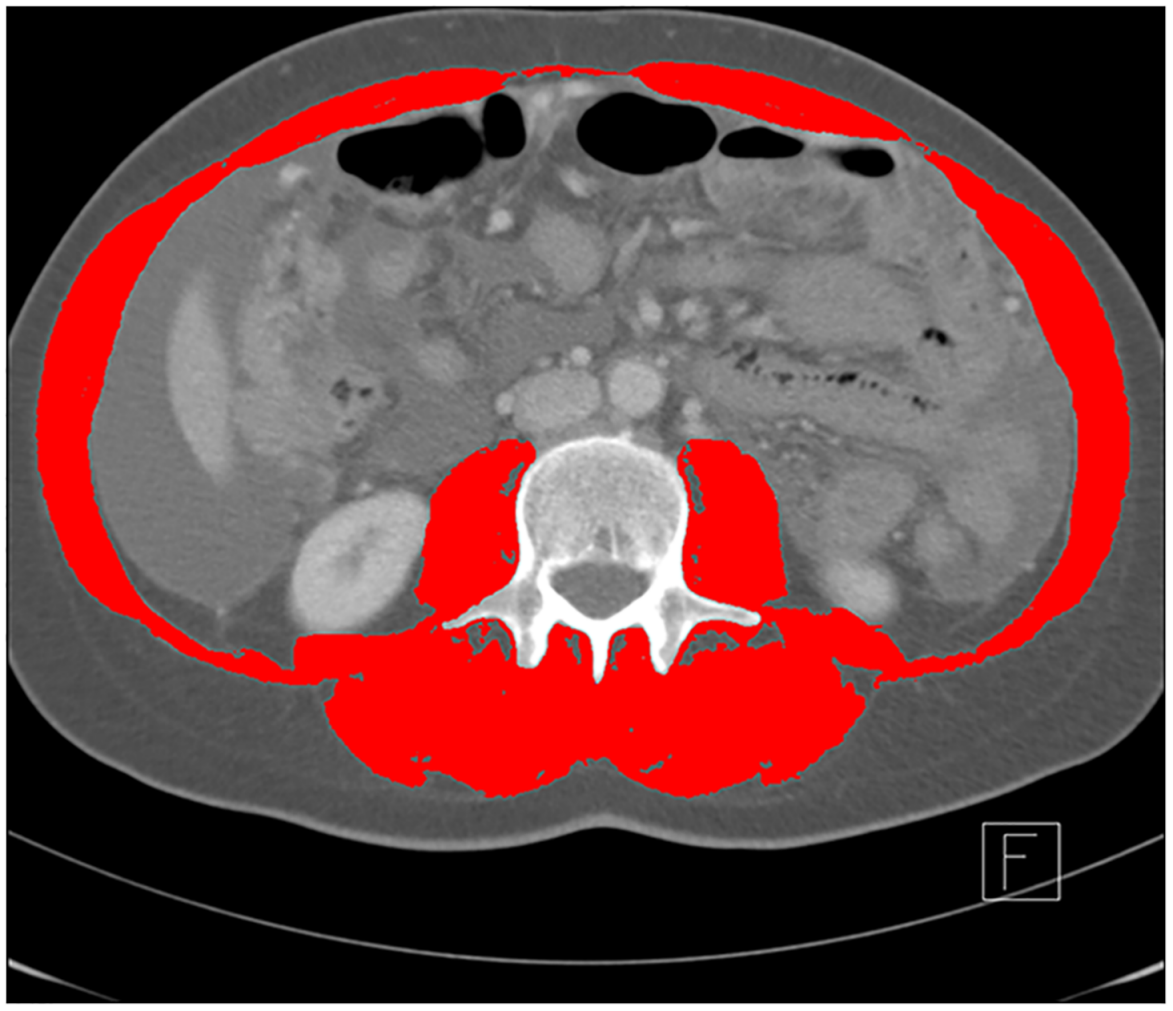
Example of an axial computed tomography (CT) image of the third lumbar vertebra region with the analyzed skeletal muscles highlighted in red.
